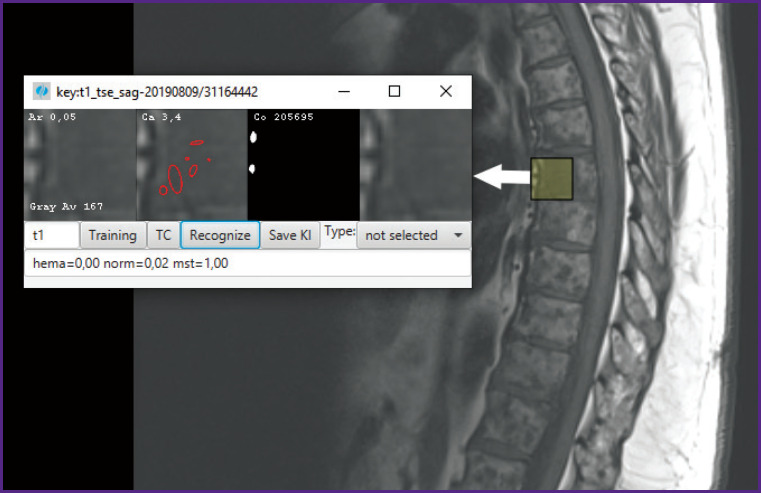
Recognition of a metastatic lesion on Th6 by sagittal data T1-weighted MRI: mst probability — 1.00
